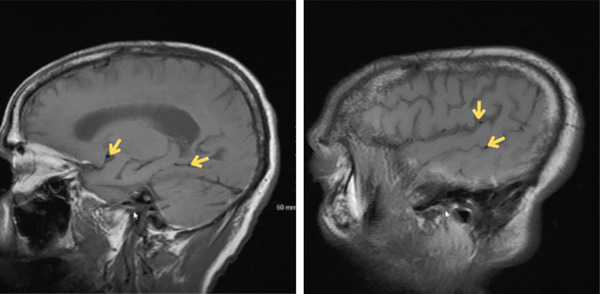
MRI of the brain showing air in the dural venous sinuses, highlighted by yellow arrows. MRI of the brain revealed air within the cerebral veins, as highlighted by yellow arrows.
